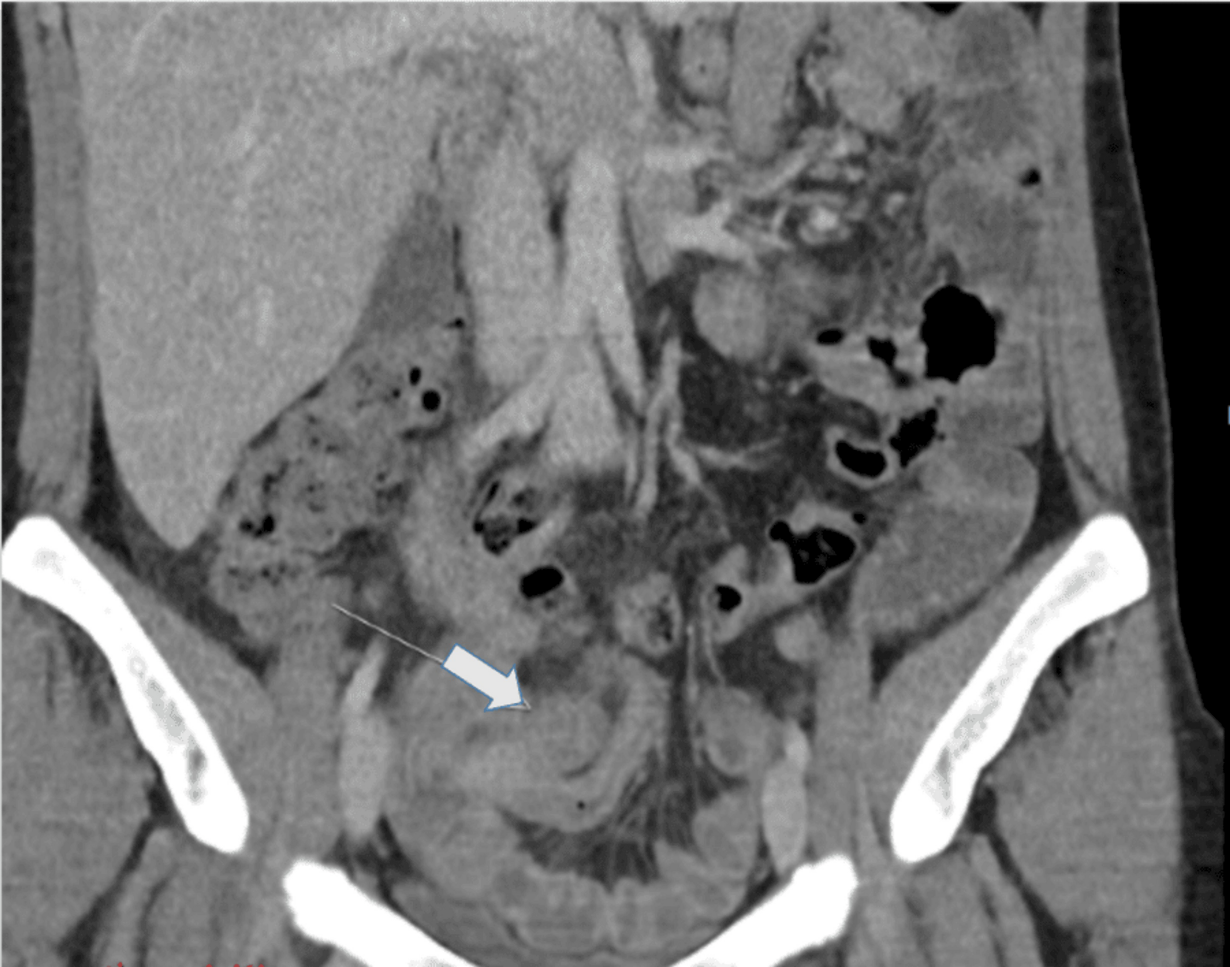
Computed tomography (CT) revealing signs of appendicitis (white arrow) Contrast-enhanced axial CT image showing an enlarged tubular structure arising from the lateral aspect of the cecum with a glove-finger appearance, consistent with an inflamed appendix. An adjacent hypodense area measuring approximately 10 × 25 mm suggests a small phlegmon or localized collection.
